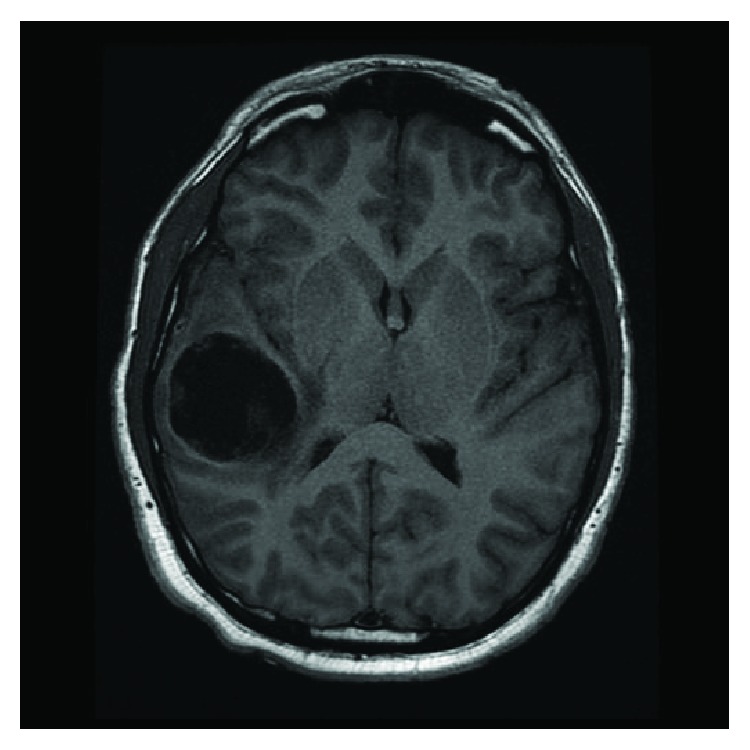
MRI showing a right temporal-parietal cystic mass that is T1 hypointense.
